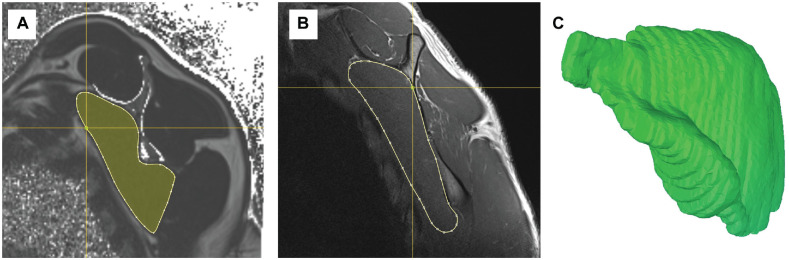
(A) Measurement of muscle fatty infiltration on sagittal magnetic resonance imaging 6-point Dixon sequences. (B) Margins of the subscapularis muscle in sectional planes (segments) of the whole cross section were manually marked on sagittal projections for assessment of muscle volume in MeVisLab. (C) The resulting 3-dimensional model presented in the Computer Assisted Surgery Planning Application (Balgrist CARD AG).
